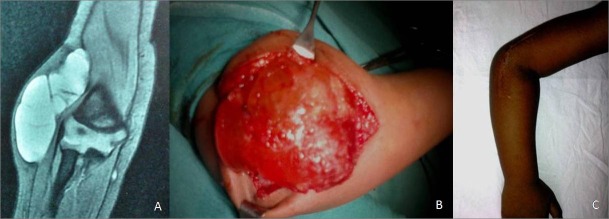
Figure 1 (A, B, C): A- MRI of elbow showing multilocular swelling with hyperintense clear signal, B-. Operative photograph showing typical findings of multilocular swelling with thin walls, C- photograph showing follow up at one year.
